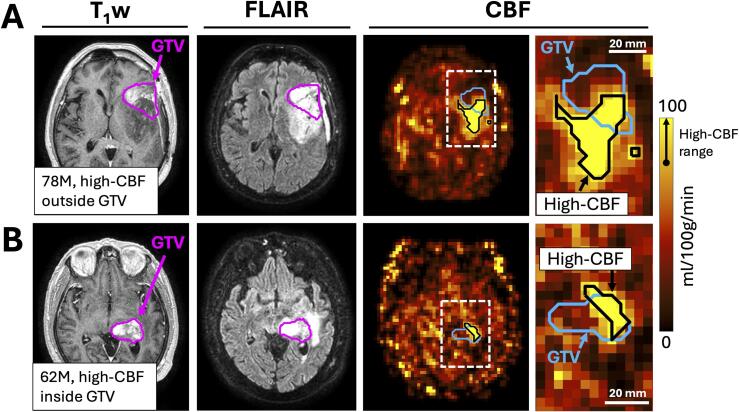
Extension of high-CBF region outside of gross tumour volume. A wide distribution was observed for the proportion of the high-CBF region which lay outside the GTV. (A): An example of a 78-year-old male patient with a large proportion of high-CBF outside the GTV (86 %). The tumour was partially resected and was MGMT unmethylated. From left to right: T_1_-weighted imaging, T_2_-FLAIR imaging, CBF map, zoomed CBF map. The GTV is shown in magenta and in blue for the anatomical images and CBF maps, respectively. The high-CBF region is shown as a black contour on the CBF map. The white dashed box over the CBF map shows the zoomed region. (B): The same set of images for a 62-year-old male patient with a small proportion of high-CBF outside the GTV (20 %). The tumour was biopsied only and was MGMT unmethylated. The color bar shows the range of values that are included in the high-CBF region (>79 ml/100 g/min). (For interpretation of the references to color in this figure legend, the reader is referred to the web version of this article.)
